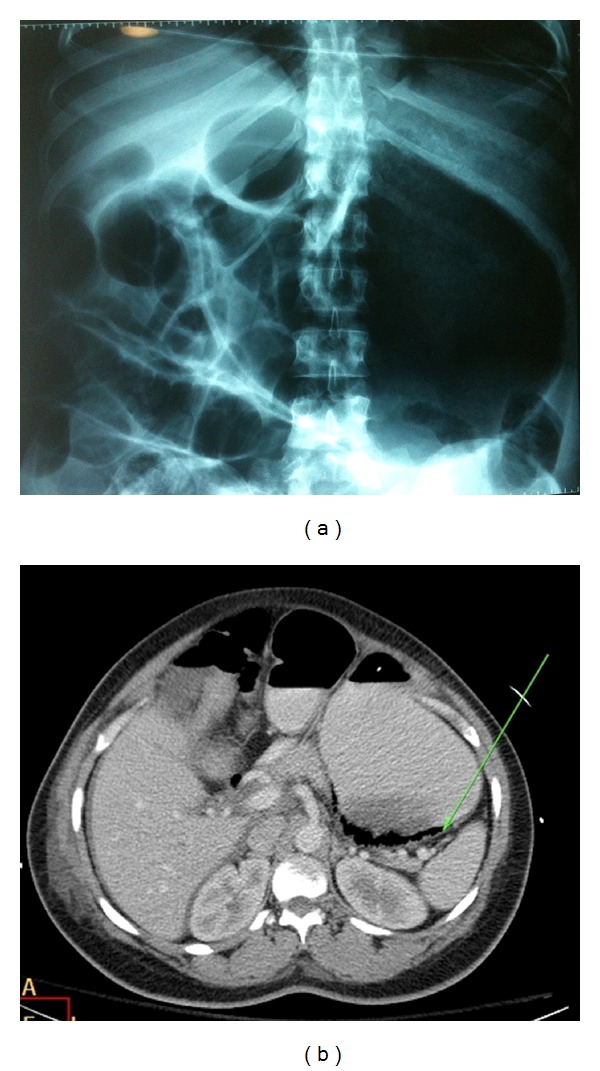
Plain film of abdomen and CT abdomen showing gastric dilatation of stomach and emphysema (arrow).
